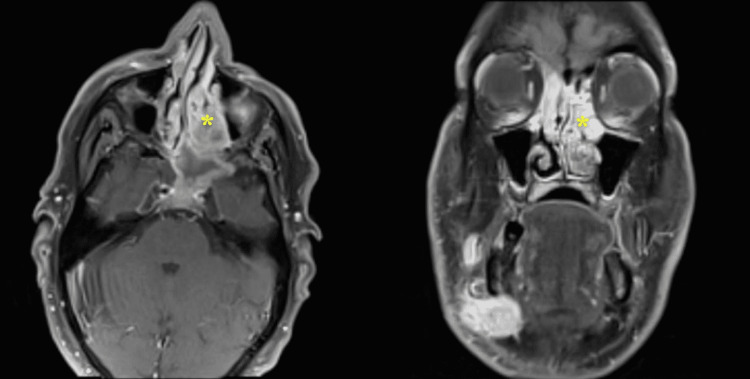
Axial and coronal MRI with contrast (T1 sequences) of the paranasal sinuses showing a homogeneous enhancing infiltrating soft-tissue mass (asterisk) with no orbital or intracranial extension.
